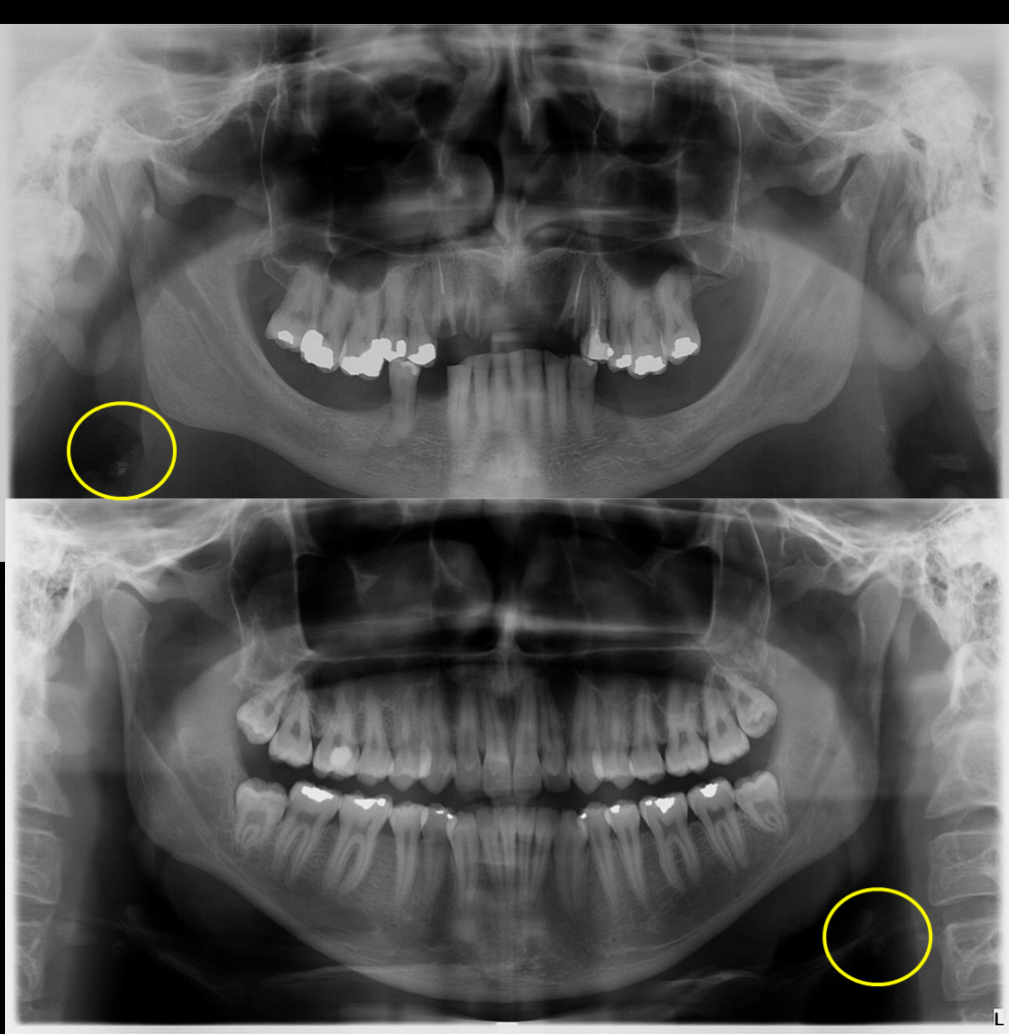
Images compatible with carotid atherosclerotic lesion detected by panoramic radiography.
